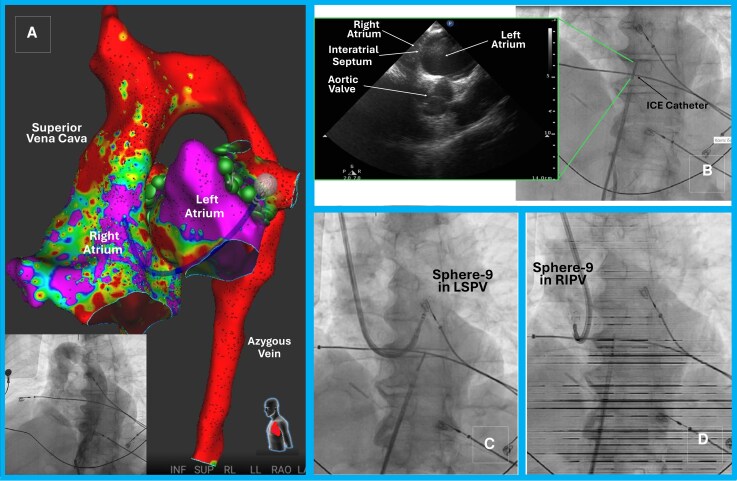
Procedural imaging and navigational approach. (*A*) Three-dimensional electroanatomical reconstruction (Affera Prism-1™) demonstrating complete inferior vena cava aplasia with venous drainage diverted via a dilated azygous vein into the superior vena cava; the inset provides a fluoroscopic angiogram confirming the anomalous venous trajectory. (*B*) Intracardiac echocardiography visualization from the azygous vein (posterior vantage point) showing the interatrial septum, fossa ovalis, and adjacent aortic valve, facilitating safe transseptal puncture from the superior approach. (*C*, *D*) Fluoroscopic views (left anterior oblique projections) showing the Sphere-9™ lattice-tip catheter positioned at the antrum of the left superior pulmonary vein and right inferior pulmonary vein via the Agilis™ steerable sheath, introduced through the right internal jugular vein.
